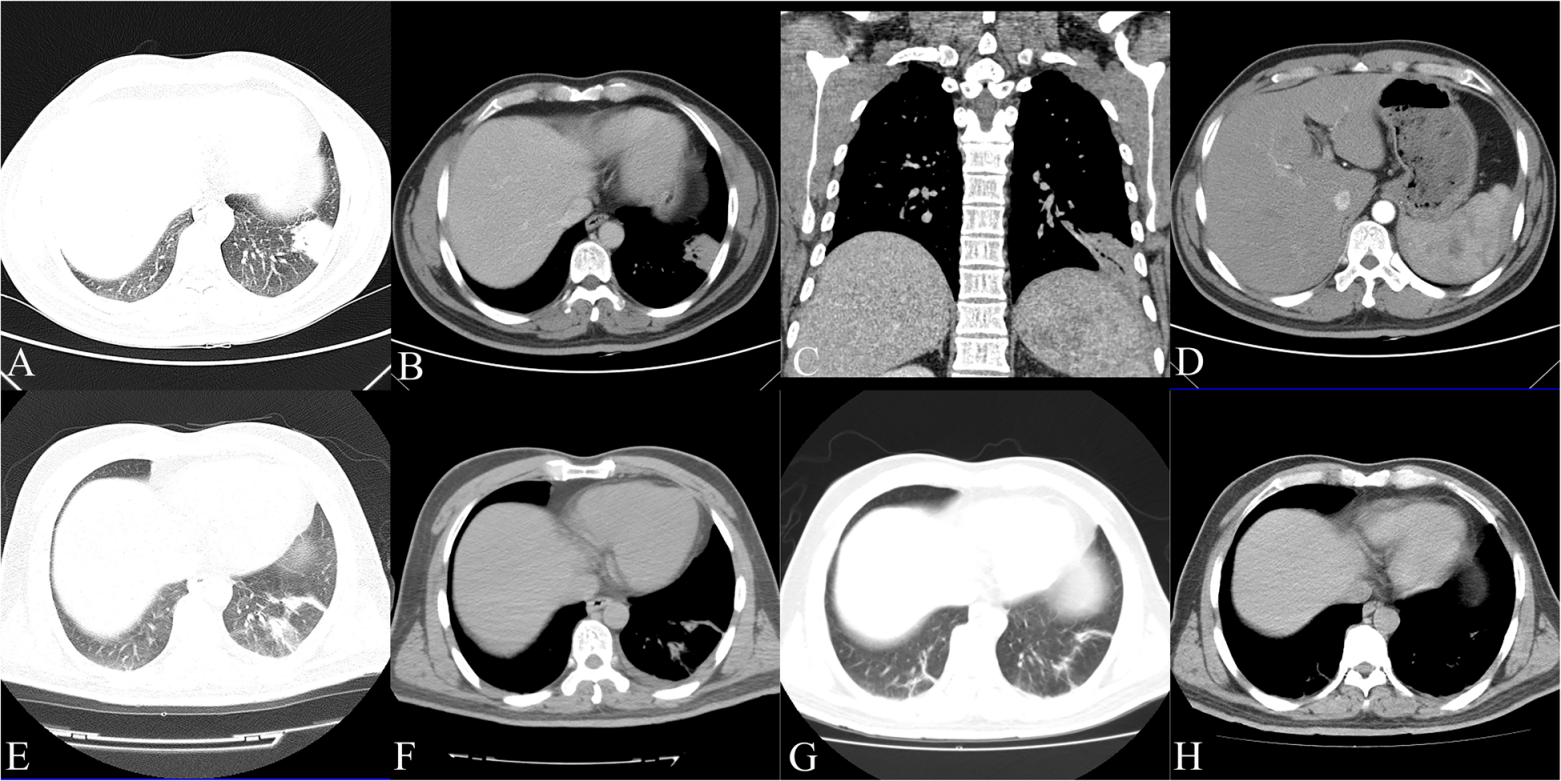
Initial chest CT (on September 11, 2015) showed a patchy opacity of 4.6 × 2.7 cm in the left lung, pleural effusion in the left chest (a, b and c), and multiple low-density lesions in the spleen (d). After a period of treatment, follow-up CT (e and f) (June 21, 2016) showed the left lung lesion had been partially, absorbed had been completely absorbed at a Aug 15, 2016, CT scan (g and h)
